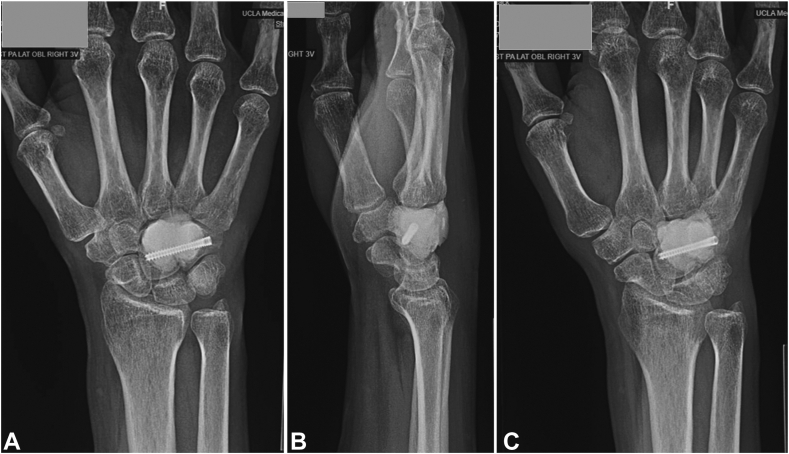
Postoperative plain radiographs status post cemented capitohamate fusion.
